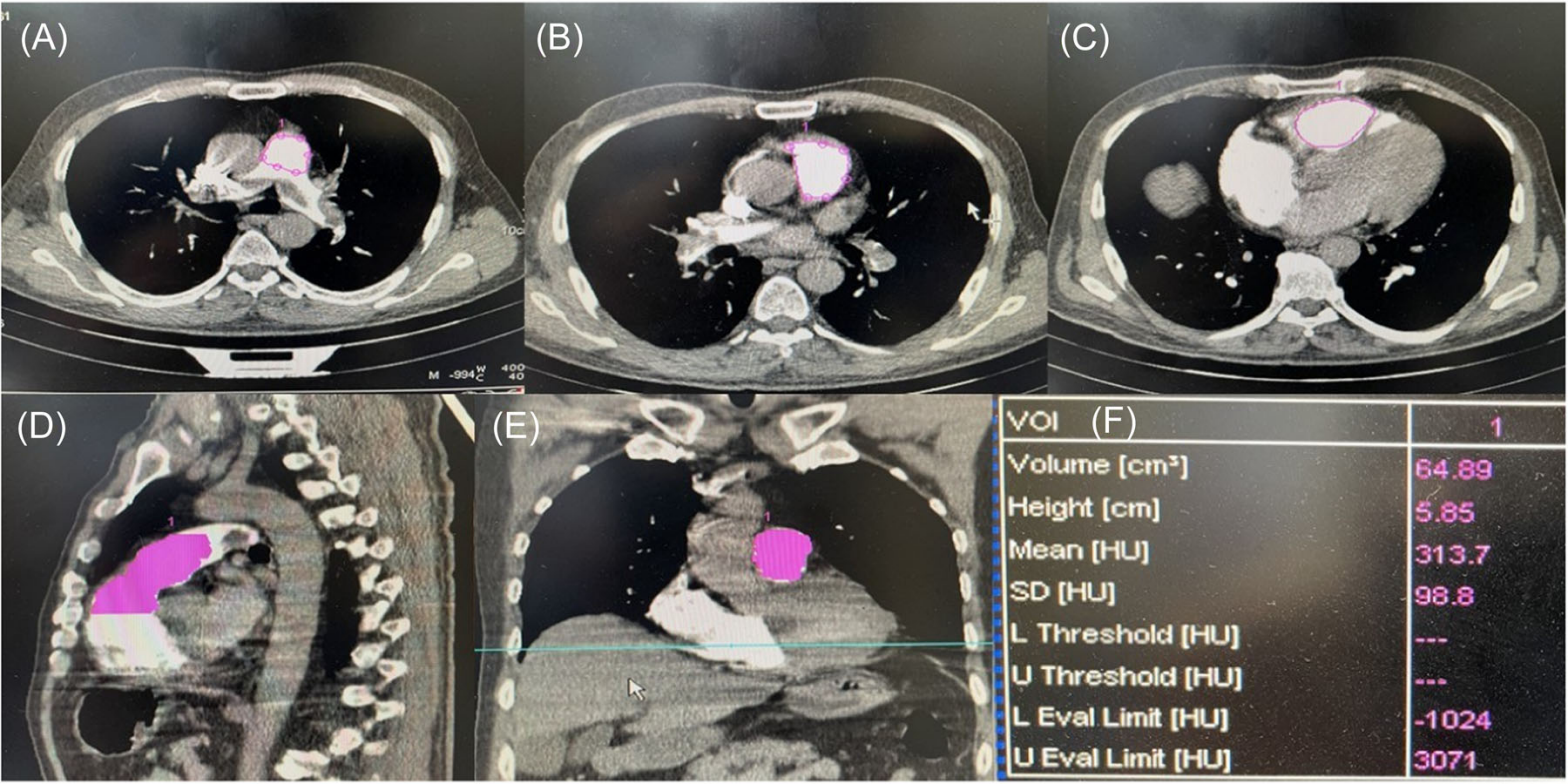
The process of evaluation of pulmonary artery trunk volume in CTPA by SOMATOM Emotion software of the CT device. In these images, axial cut of pulmonary artery trunk is shown in in three planes of pulmonary bifurcation (A), median part of pulmonary trunk (B), and the initial part of pulmonary trunk just after the tricuspid valve (C) which are delignated by pink lines. The software calculates the pulmonary trunk volume the marked lines (D and E) and generates the values in a table (F).
